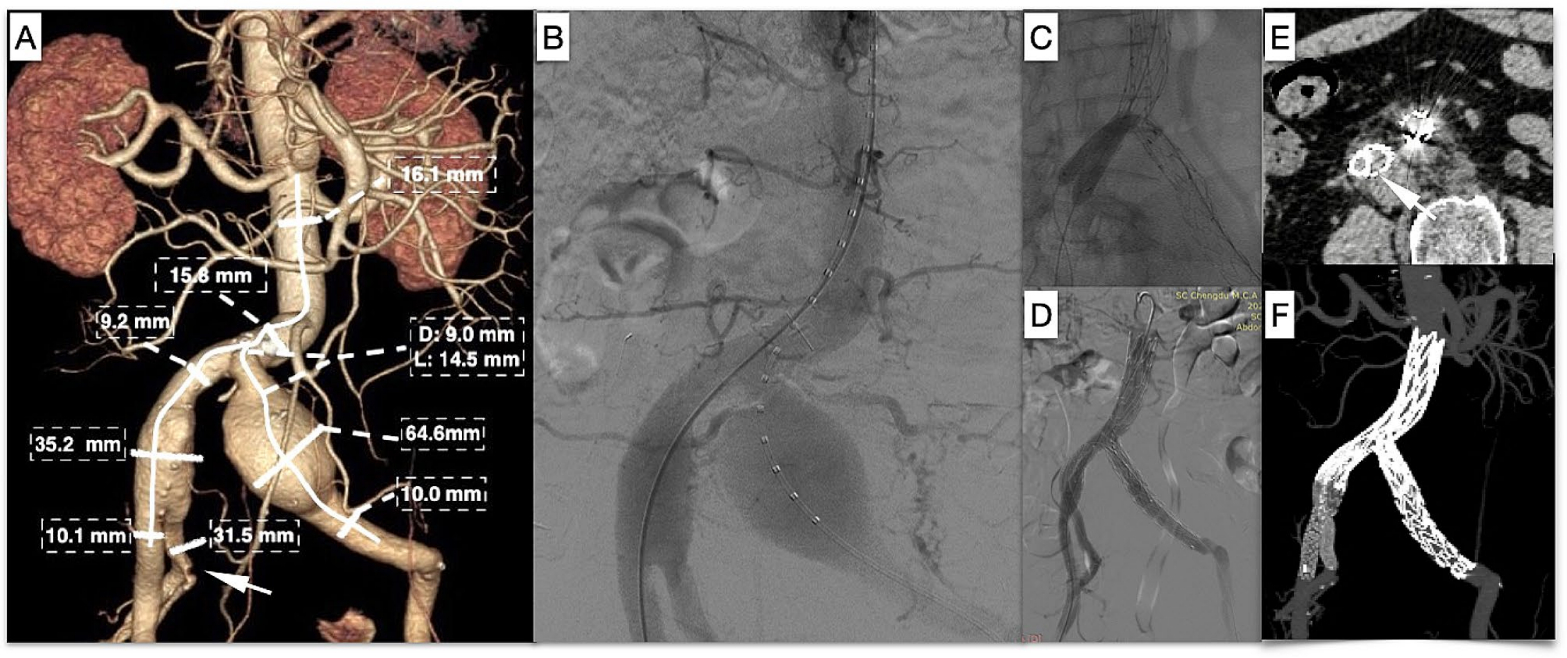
Case 2: A Preoperative CT imaging and aneurysm anatomical parameter measurements. The right proximal CIA measured 9.2 mm in diameter, the left proximal CIA measured 9.0 mm in diameter, and the length of the nonaneurysmal proximal CIA was 14.5 mm. B Intraoperative angiogram. C Two noncompliant 10 mm balloons were simultaneously inflated inside the parallel covered stents. D The final angiogram demonstrated successful deployment of Aegis™ and two parallel covered stents in the right iliac artery. E The cross-sectional image of the two-year follow-up CT scan demonstrated the “eye of the tiger” configuration (white arrow), with no evidence of gutter leaks. F CT scan three-dimensional reconstruction
